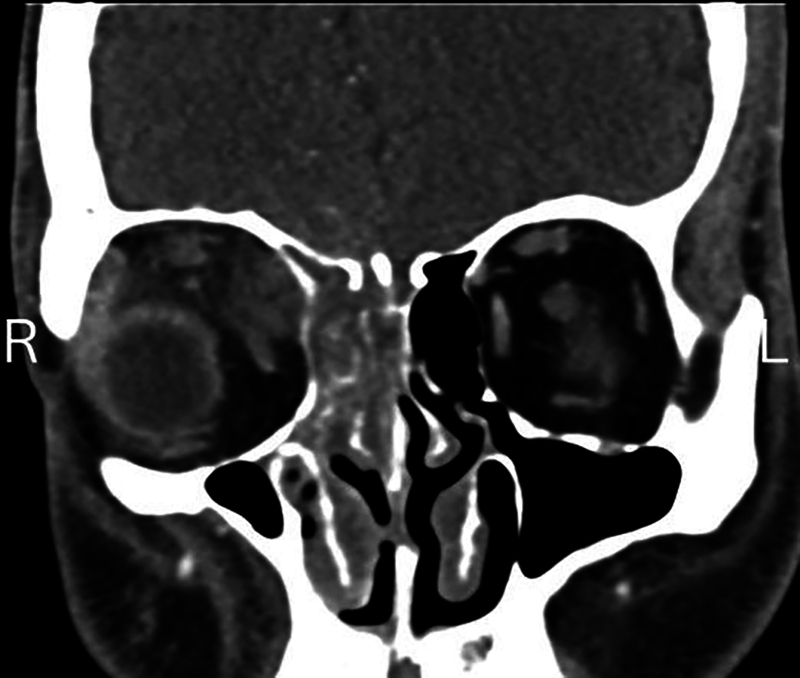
Preoperative coronal CT showing the sinonasal involvement of the infection.
